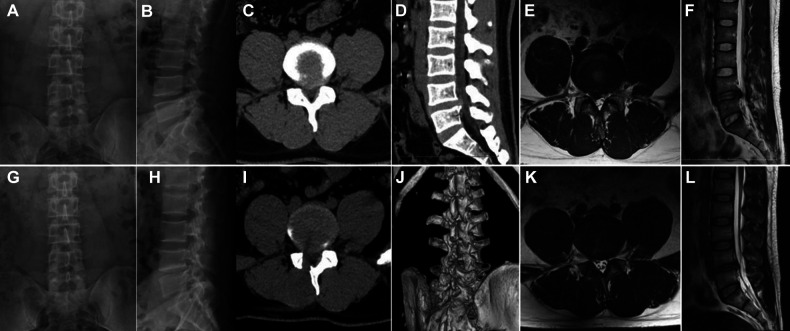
Pre- and postoperative x-ray, CT and MRI of UBE. (A,B) Preoperative x-ray. (C–F) Preoperative CT and MRI showing disc herniation. (G,H) Postoperative x-ray. (H,I) Postoperative CT and MRI showing the extruded disc was removed.
